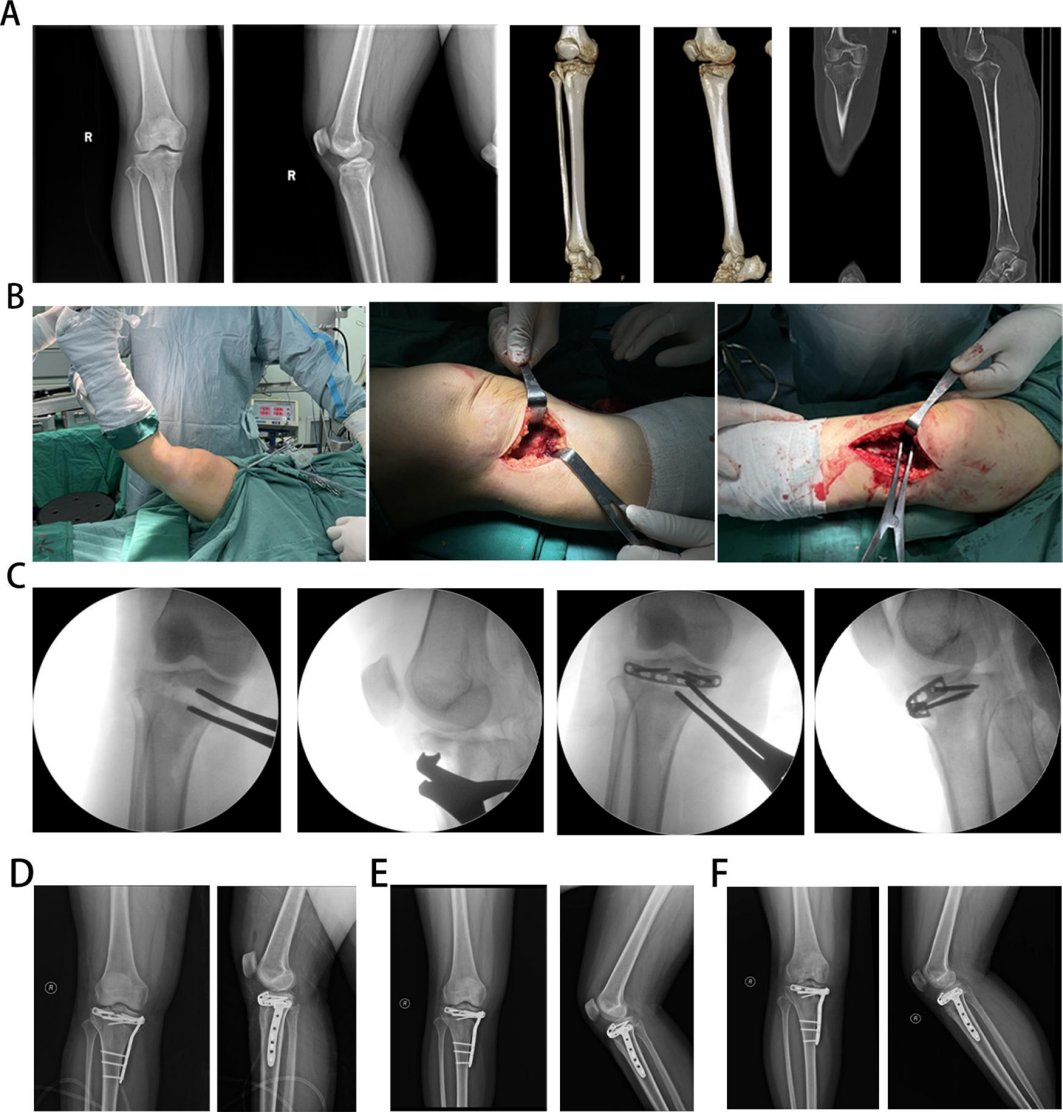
Typical surgical case images: (A) A 40-year-old female patient presented with bicondylar HTPF; preoperative x-ray image of patients; x-ray images of patients; preoperative 3-dimensional computed tomography imaging of patients; (B) intraoperative images of the surgical approach; (C)intraoperative C-arm fluoroscopy images. (D) x-ray image of patients 1 month after surgery; (D) x-ray image of patients 3 months after surgery.(F) x-ray image of patients 12 months after surgery.
